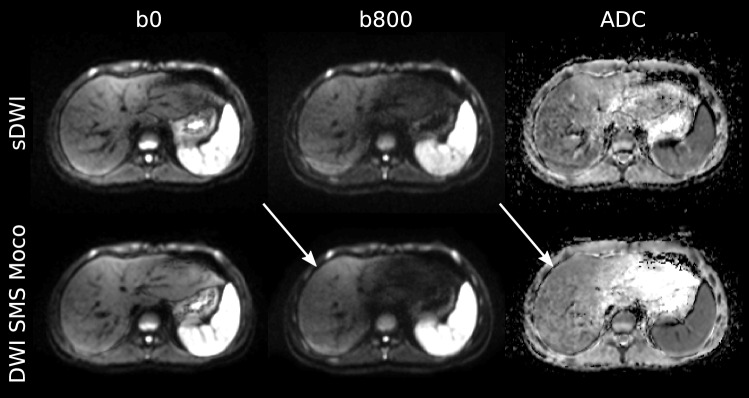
Example of an adolescent patient. 14-year-old male patient with CF, cystic fibrosis related diabetes (CFRD) and cystic fibrosis related liver disease (CFRLD). Images show the acquired DWI images with *b*-values of 0 (b0) and 800 (b800) as well as the corresponding ADC map for both acquisitions of sDWI and DWI SMS Moco. Improved image quality with homogeneous image impression of the liver parenchyma in the b800 image and ADC map in DWI SMS Moco compared to standard DWI (white arrows)
